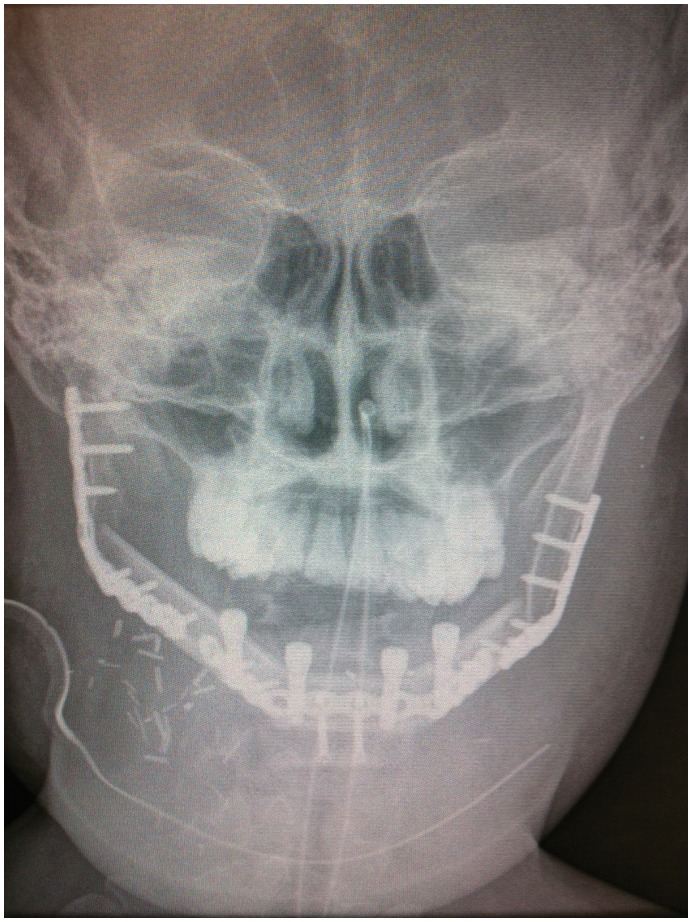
Postoperative x-ray, demonstrating good bony contour, with reconstruction plates used for mandibular fixation, screws used for genioplasty and osseointegrated dental implants inserted primarily.
